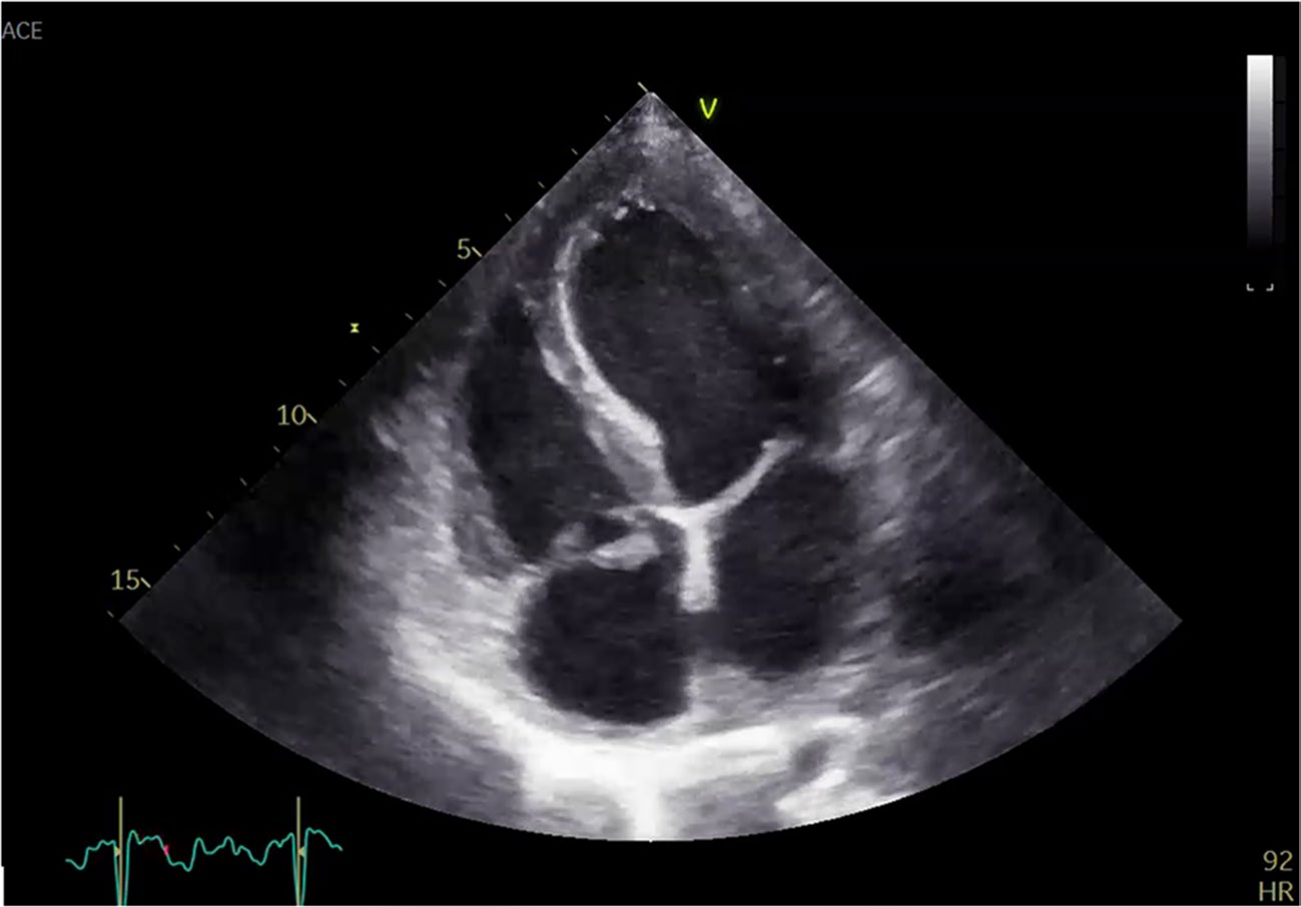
Transthoracal echocardiography of a tricuspid valve endocarditis
